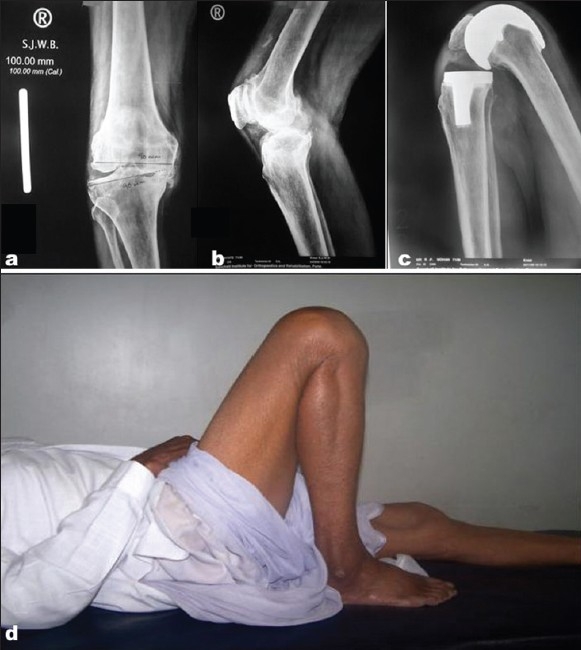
Pre-operative radiographs antero-posterior view (a) and lateral view (b) of the knee of 71 years old patient shows severe OA with genu varum. Post-operative lateral radiograph (c) show range of flexion achieved and clinical photographs (d) shows the range of flexion at 28 months follow-up
